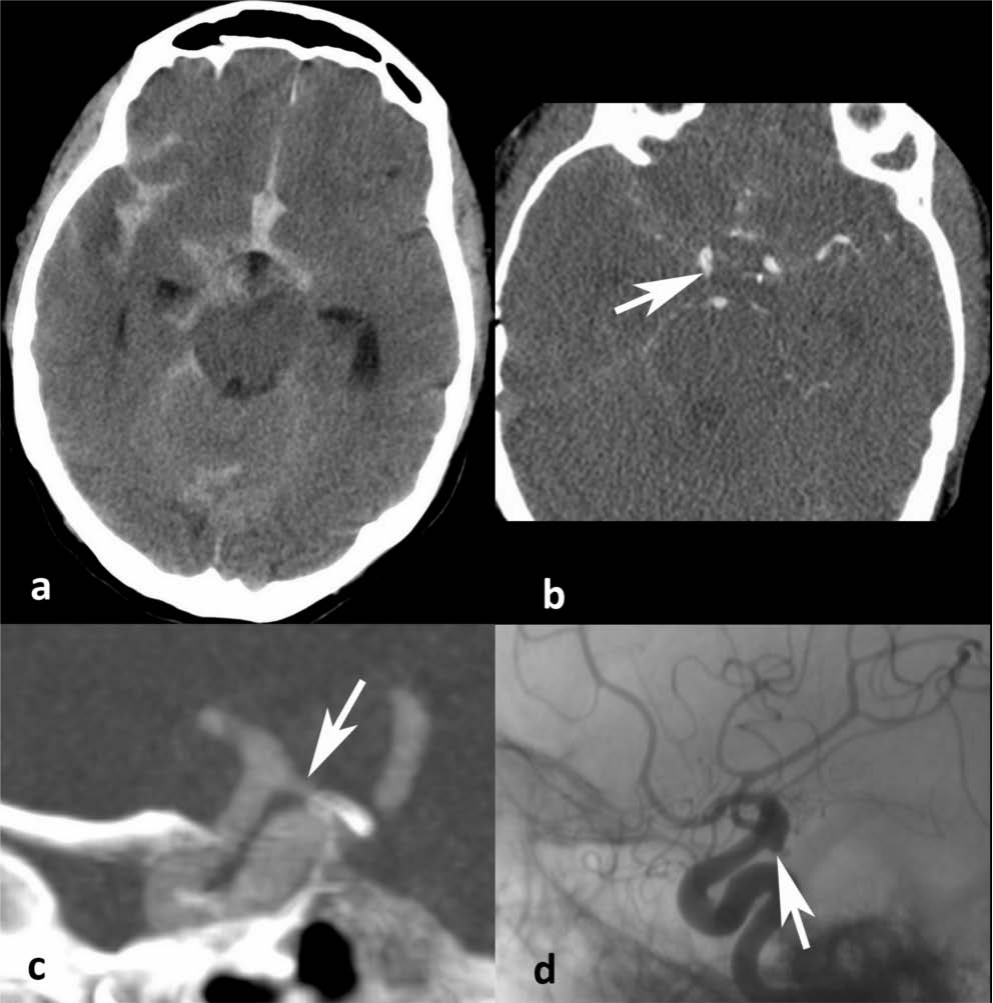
Missed aneurysm. Aneurysm missed by each neuroradiologist on the CT Angiography (CTA). a Non-contrast CT displaying the aneurismal SAH pattern. b CTA showing the posterior communicating aneurysm (arrow). c 3D reconstruction of the CTA showing the aneurysm filled with contrast material, appearing to be continuous with the sphenoid bone (arrow). d Angiogram of the same 3 mm aneurysm on the posterior communicating artery (arrow)
